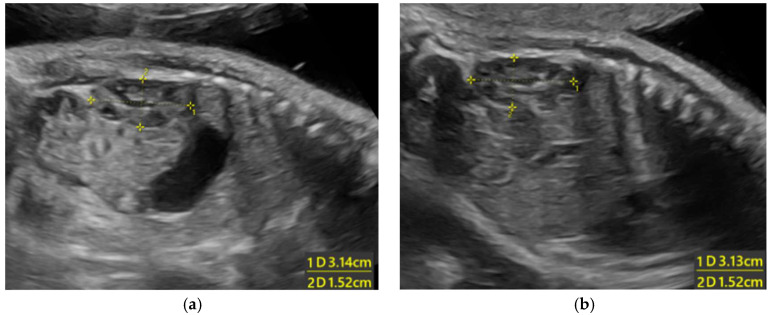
Ultrasonographic images of small kidneys (length of the kidneys are below the 3rd percentile for 34 weeks weeks) [20]: (a) left kidney; (b) right kidney.
